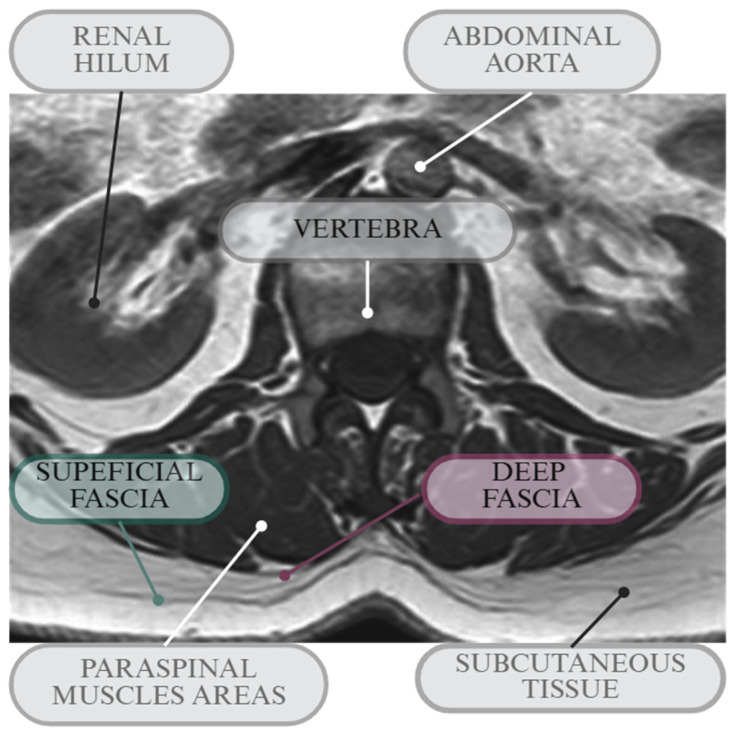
Example MRI (axial, T2-weighted) with the relative anatomical structures (created in BioRender. Bonaldi, L. (2025) https://BioRender.com/n2jgy4l).
